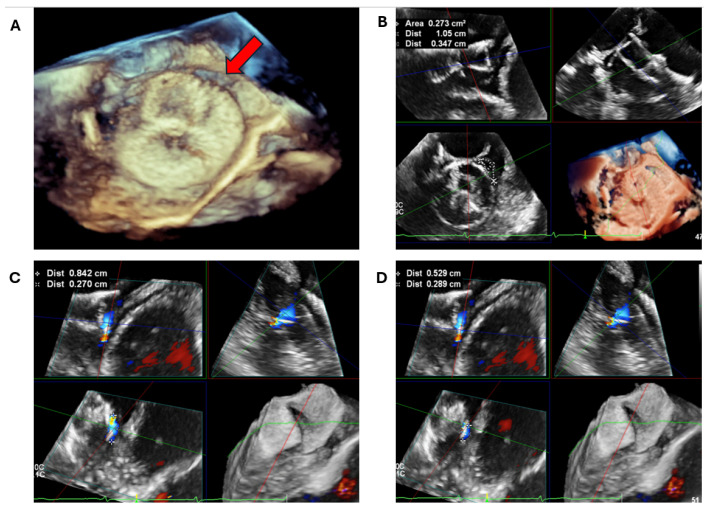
The same patient in Figure 16 was assessed with TOE. The antero-superior PDL tract can be visualised on the 3D zoom image (red arrow) (A). This measures 3 × 11 mm with a cross-sectional area of 0.3 cm^2^ at the level of the disc on 3D multiplanar analysis (B). On 3D colour doppler analysis, high-velocity flow can be visualised into the distal LAA, which measures 3 × 8 mm at the level of the mid-lobe (C) and 3 × 5 mm at the level of the distal lobe (D). TOE: Transoesophageal echocardiogram; 3D: Three-dimensional; PDL: Peri-device leak; LAA: Left atrial appendage.
